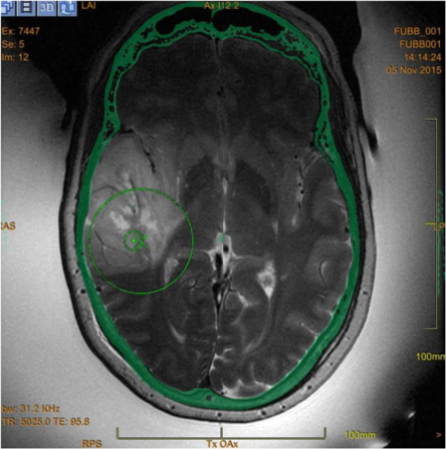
Intraoperative T2w MR image showing the tumor and the first BBB target
